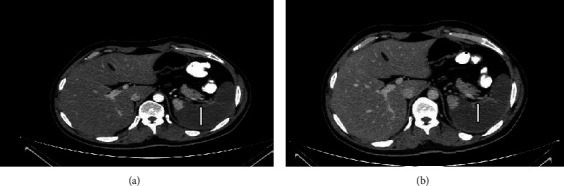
Abdominal CT shows artery occlusion and complete absence of blood circulation on the spleen. White arrow shows the infract after the enhancement of contrast material at the (a) arterial and (b) venous phase.
